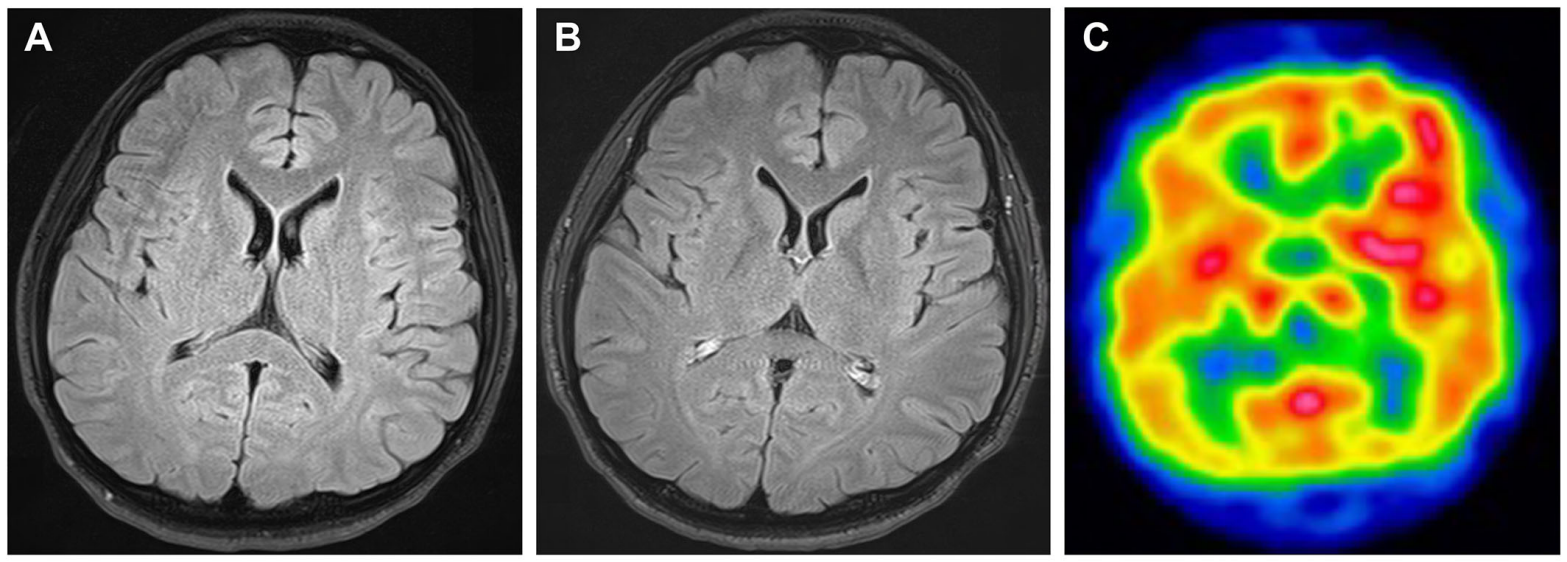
Brain images taken prior to immunotherapy. No abnormalities are obvious in fluid-attenuation inversion recovery (FLAIR) magnetic resonance imaging (A) or contrast-enhanced FLAIR imaging (B). Brain single-photon emission computed tomography displayed increased 99mTc-ECD uptake in the left frontotemporal region (C).
